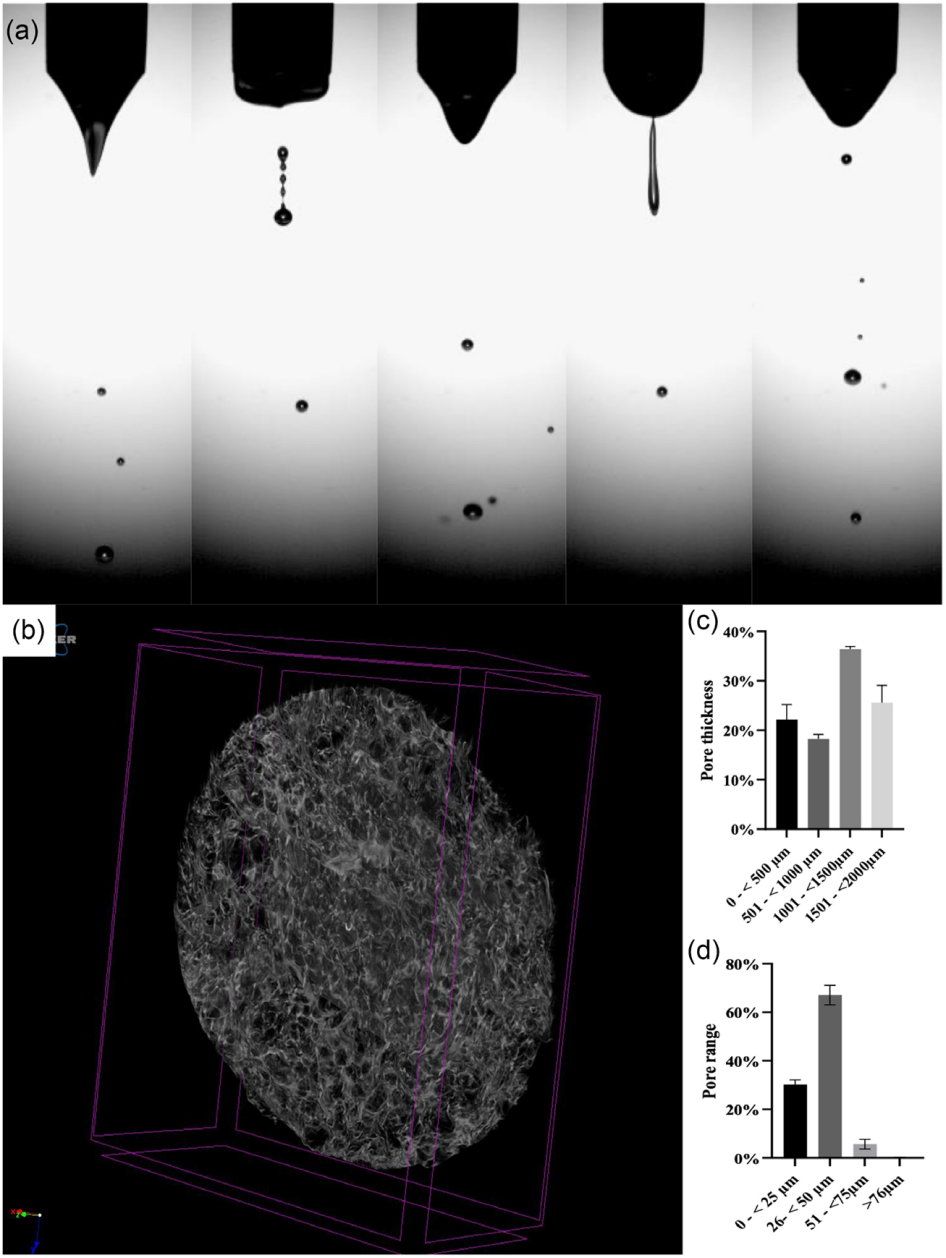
a) Representative digital high‐speed image sequence of the electrospraying of the collagen solution for scaffolding captured at 15 000 fps, b) characteristic micro‐CT of the 6 mm biopsy punched scaffold generated via electrosprays. Panels c,d) demonstrate scaffold characteristics in particular pore thickness and pore range, respectively.
